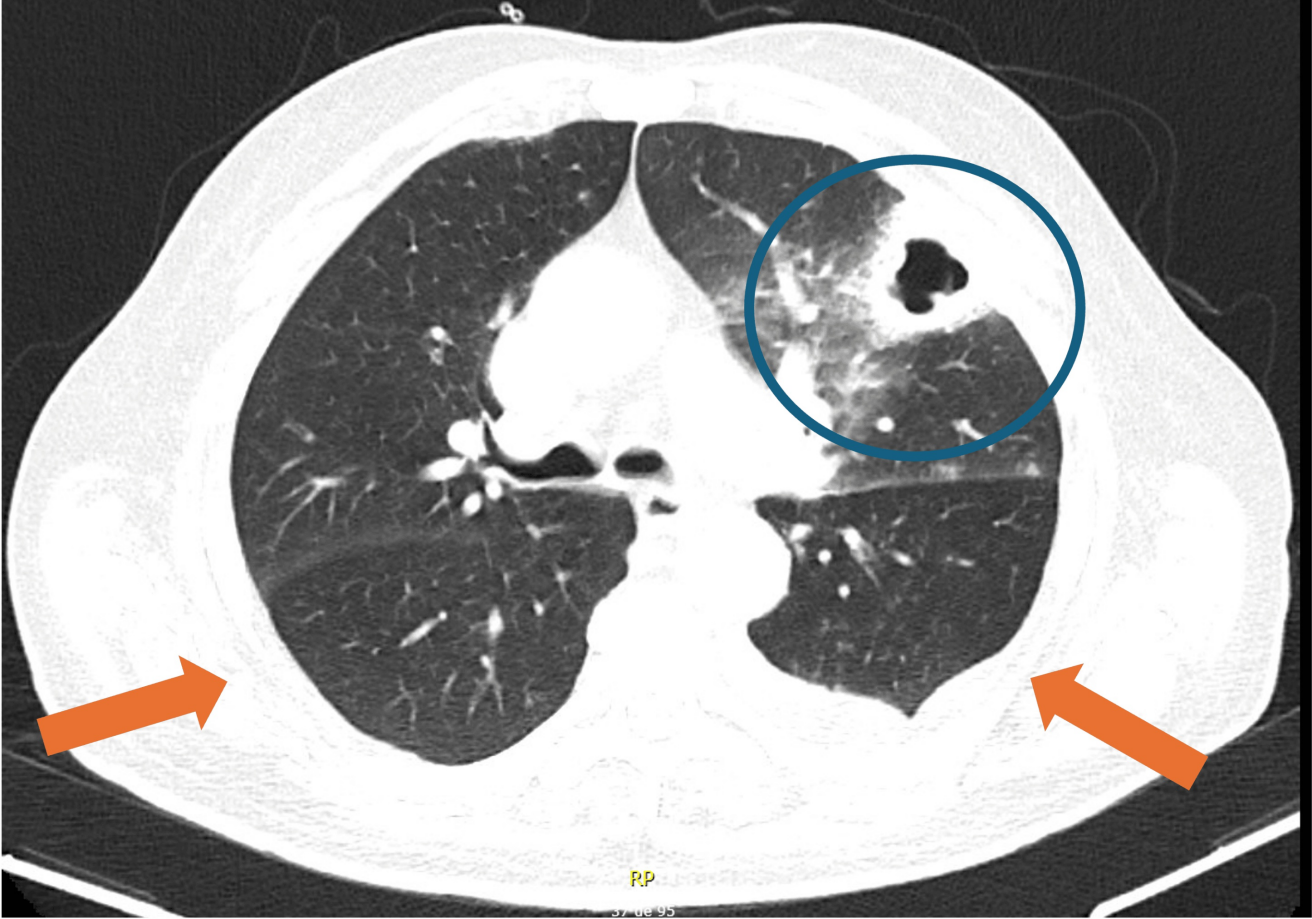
Axial Chest CT showing bilateral progression with cavitary transformation (blue circle) and pleural effusion (orange arrows)
